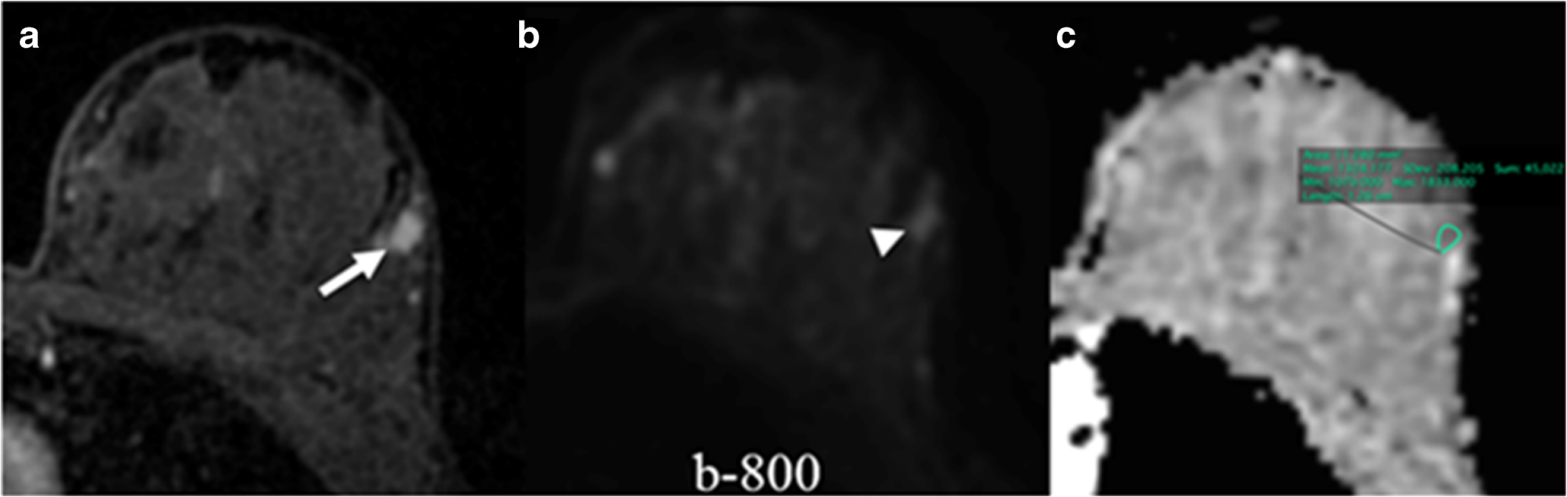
A case of a false negative in DWI. Axial images of a biopsy-proven DCIS. Contrast-enhanced image (a) shows a 7-mm oval irregular enhancing lesion (arrow) in the left breast. Acquired DWI b-800 (b) shows an oval apparently restricting lesion (arrowhead). The ADC map (c) shows a region of interest drawn in the corresponding location which yields an ADC mean value of 1324 × 10^−6^ mm^2^/s. This lesion was correctly categorized as malignant in DCE-MRI but categorized as benign in DWI. DWI, diffusion-weighted imaging; DCIS, ductal carcinoma in situ; ADC, apparent diffusion coefficient; DCE-MRI, dynamic contrast-enhanced magnetic resonance imaging
